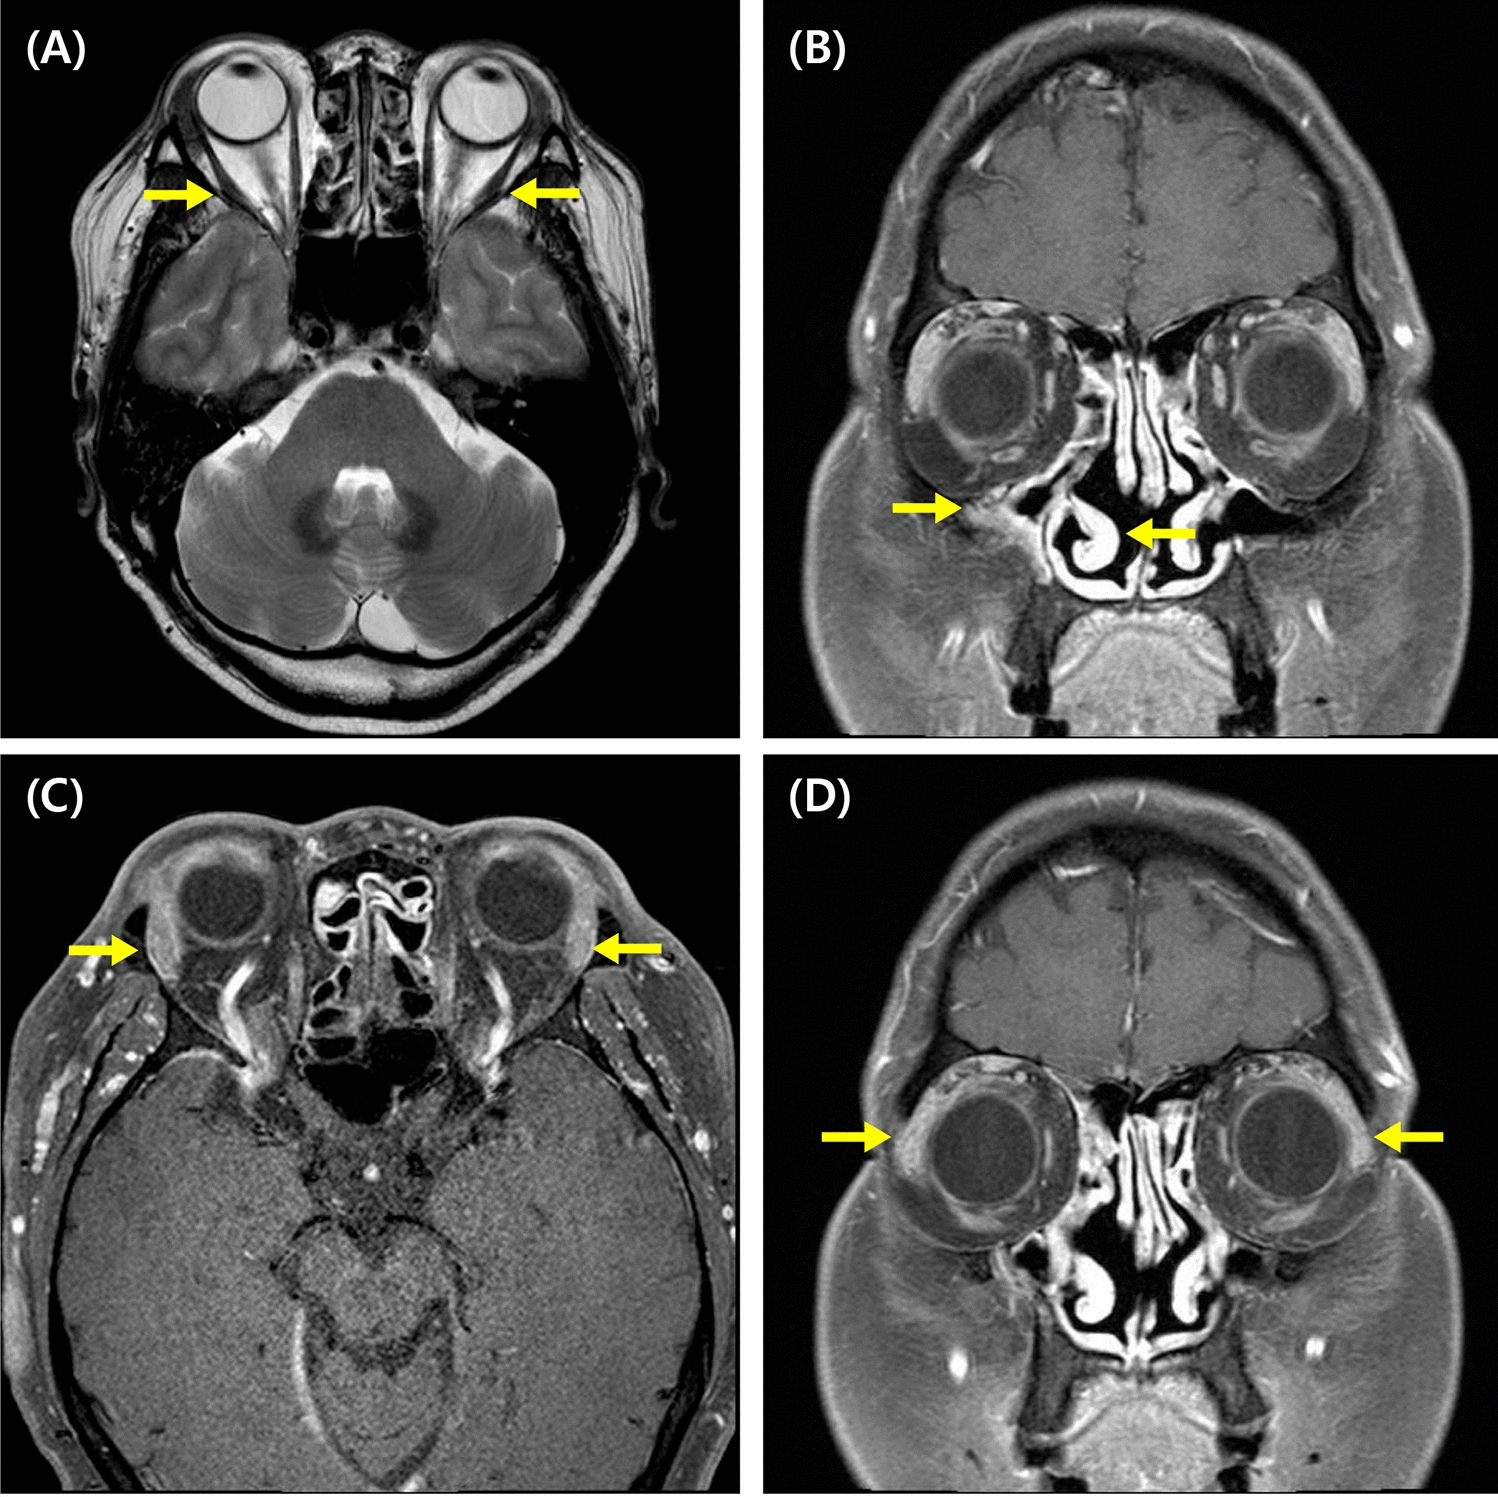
Magnetic resonance imaging of the patient before dupilumab treatment. A Bilateral lateral rectus muscle enhancement, B right maxillary sinusitis, C, D bilaterally enlarged and enhanced lacrimal glands. The corresponding lesions are marked by yellow arrows
